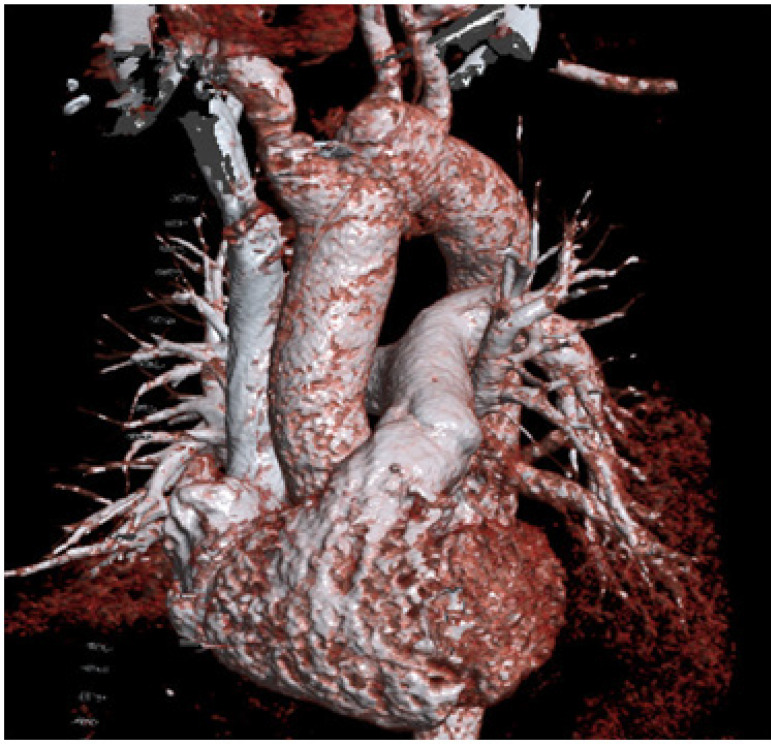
3D reconstruction of postoperative CT showing the replacement of the ascending aorta and the re-implanted brachiocephalic trunk.
